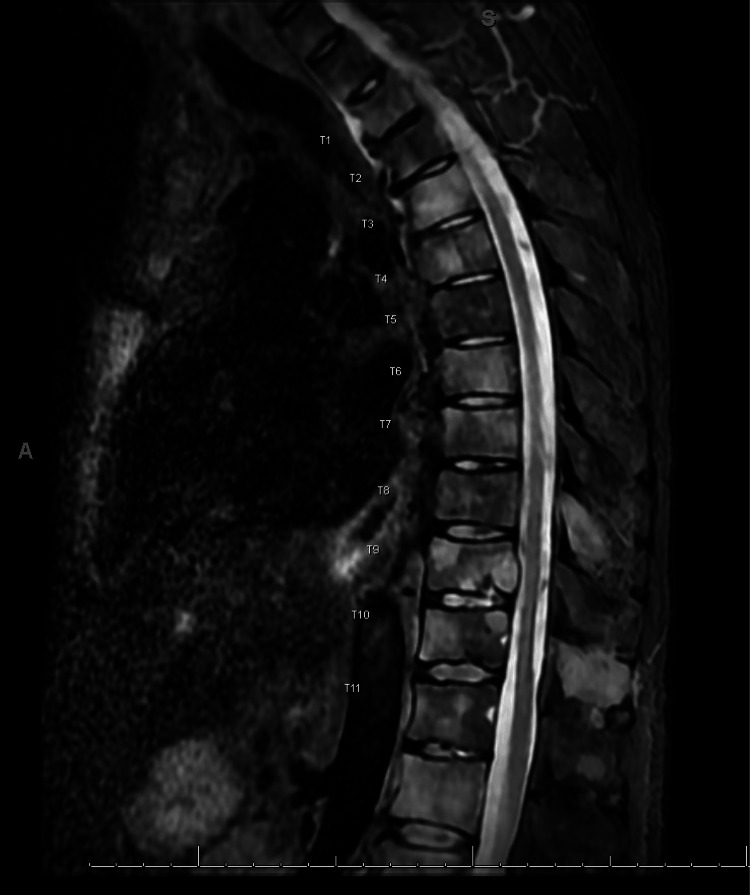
MRI T spine sagittal view with contrast showing extensive abnormal enhancement throughout the osseous structures compatible with metastases
